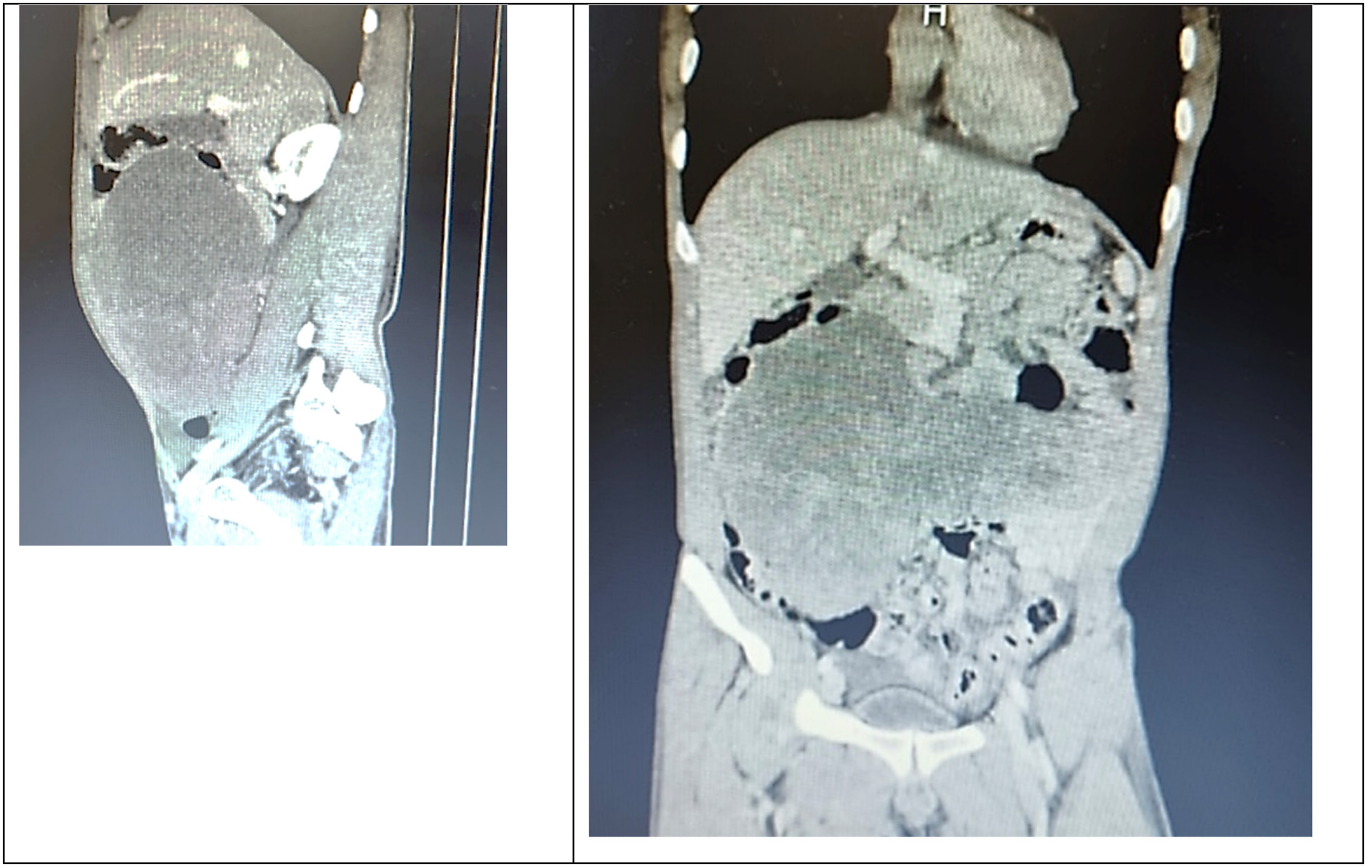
Contrast‐enhanced computed tomography images show huge soft tissue attenuation, heterogeneously enhancing intraperitoneal mass in the right mid, central, left mid, and right lower abdomen.
